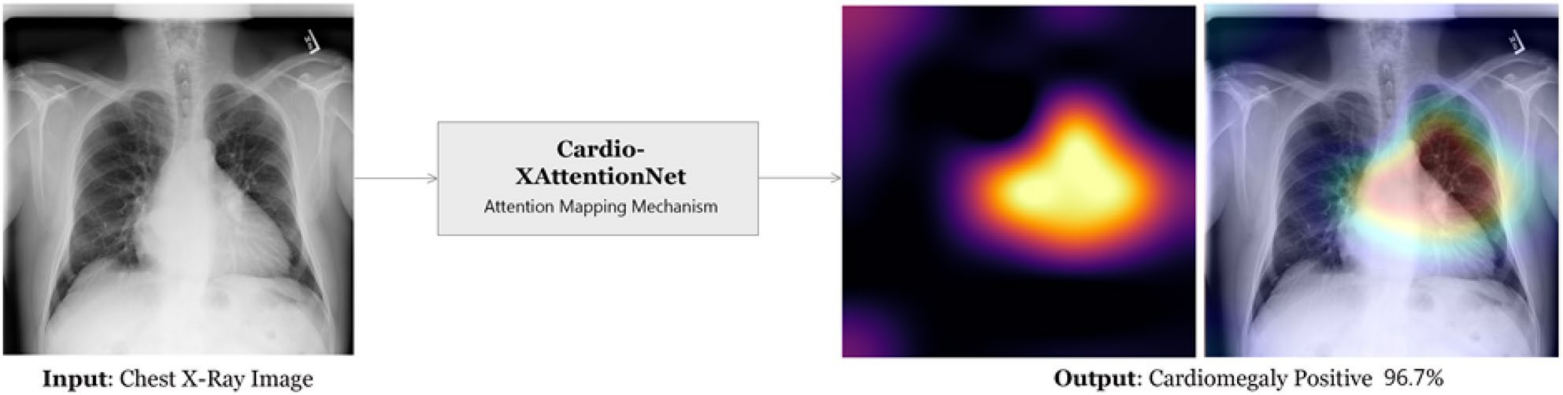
Cardio-XAttentionNet (CXA_Dense121) model, based on DenseNet-121 with Attention Mapping Mechanism, generating accurately positive outcomes with the most indicative region mapping for cardiomegaly, addressing class information and localization on the frontal-view chest X-Ray image. On left side, the original image. On right side, the attention map, and the superimposed outcomes with intensity factor 0.2.
